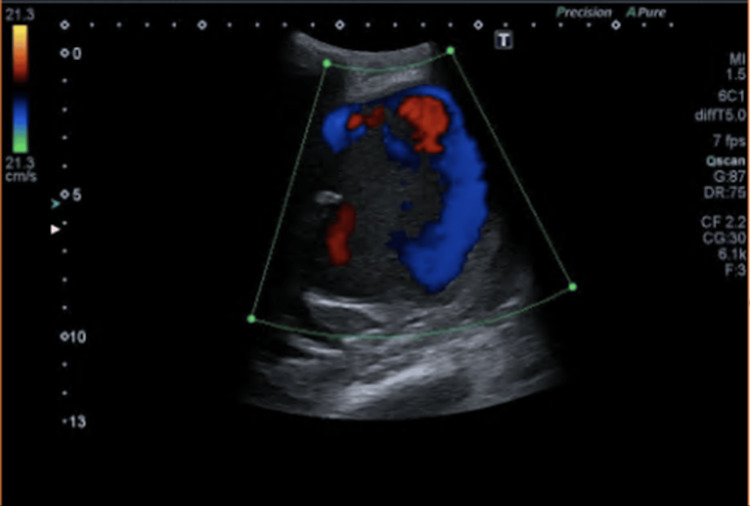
Abdominal-pelvic Doppler ultrasound showing a yin-yang sign.
